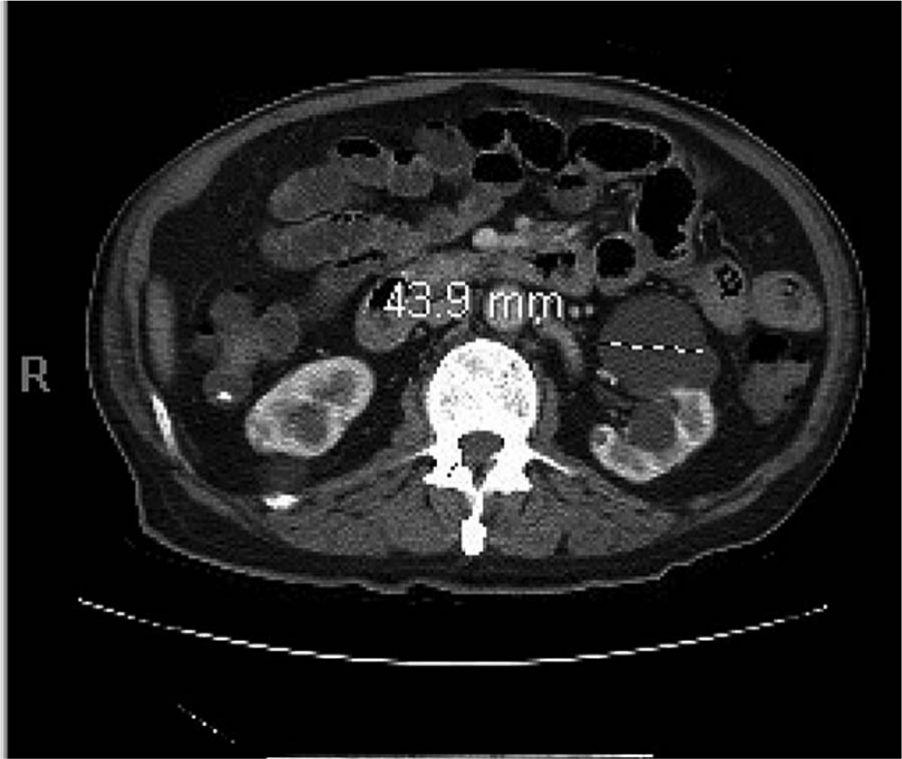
Abdominal CT scan showing thickened jejunal loops in the right upper quadrant, suggestive of small bowel involvement in eosinophilic gastroenteritis.
